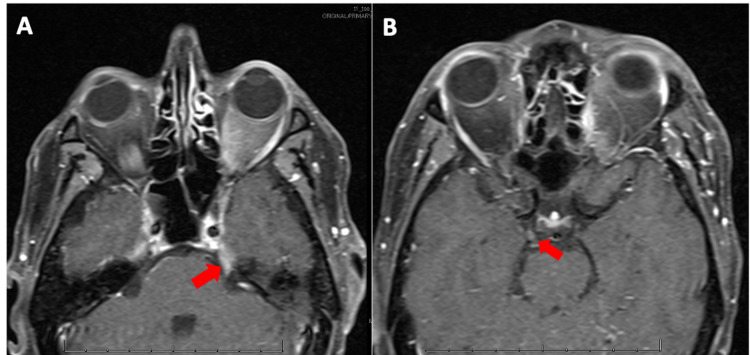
Axial T1 post-contrast MRI images. (A) Red arrow pointing to abnormal enhancement of the cisternal segment of the left trigeminal nerve. (B) Red arrow pointing to abnormal enhancement involving the distal cisternal and cavernous segment of the right oculomotor nerve.
